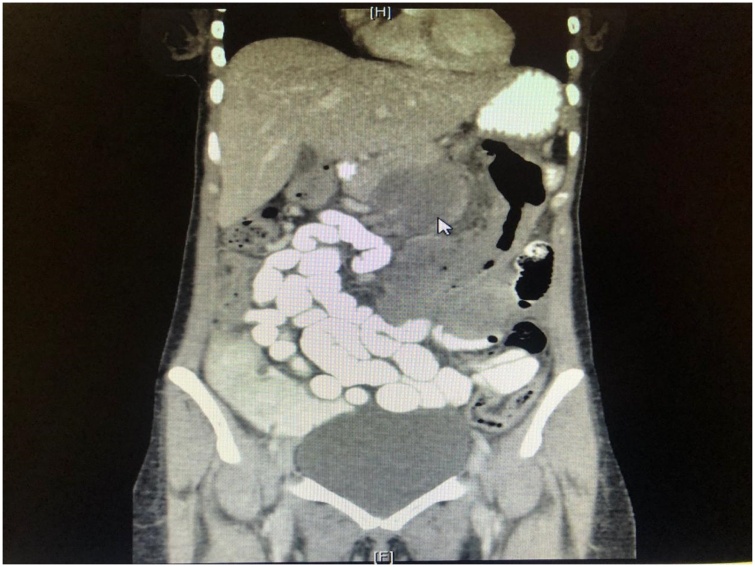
Coronal enhanced computed tomography scan of the abdomen showing an obstructing transverse colon mass (arrow).
